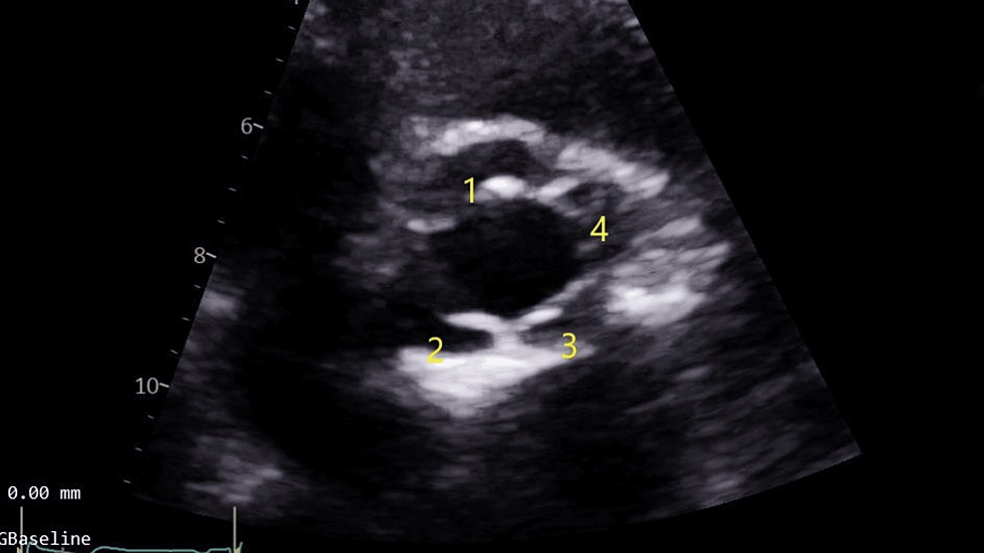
Transthoracic echocardiogram, systolic view
